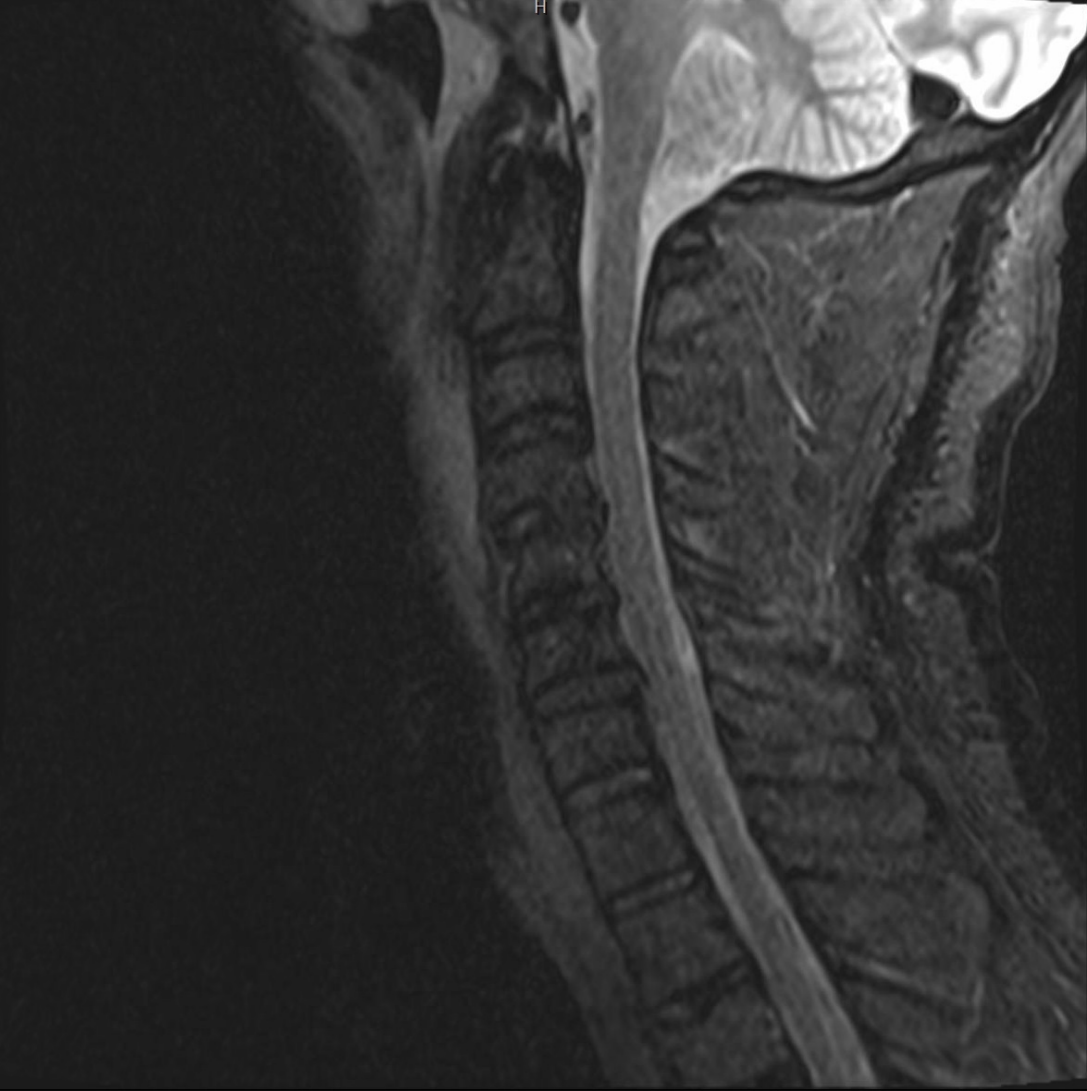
Magnetic Resonance Imaging of the Cervical Spine Sagittal cervical spine short tau inversion recovery (STIR) sequence
